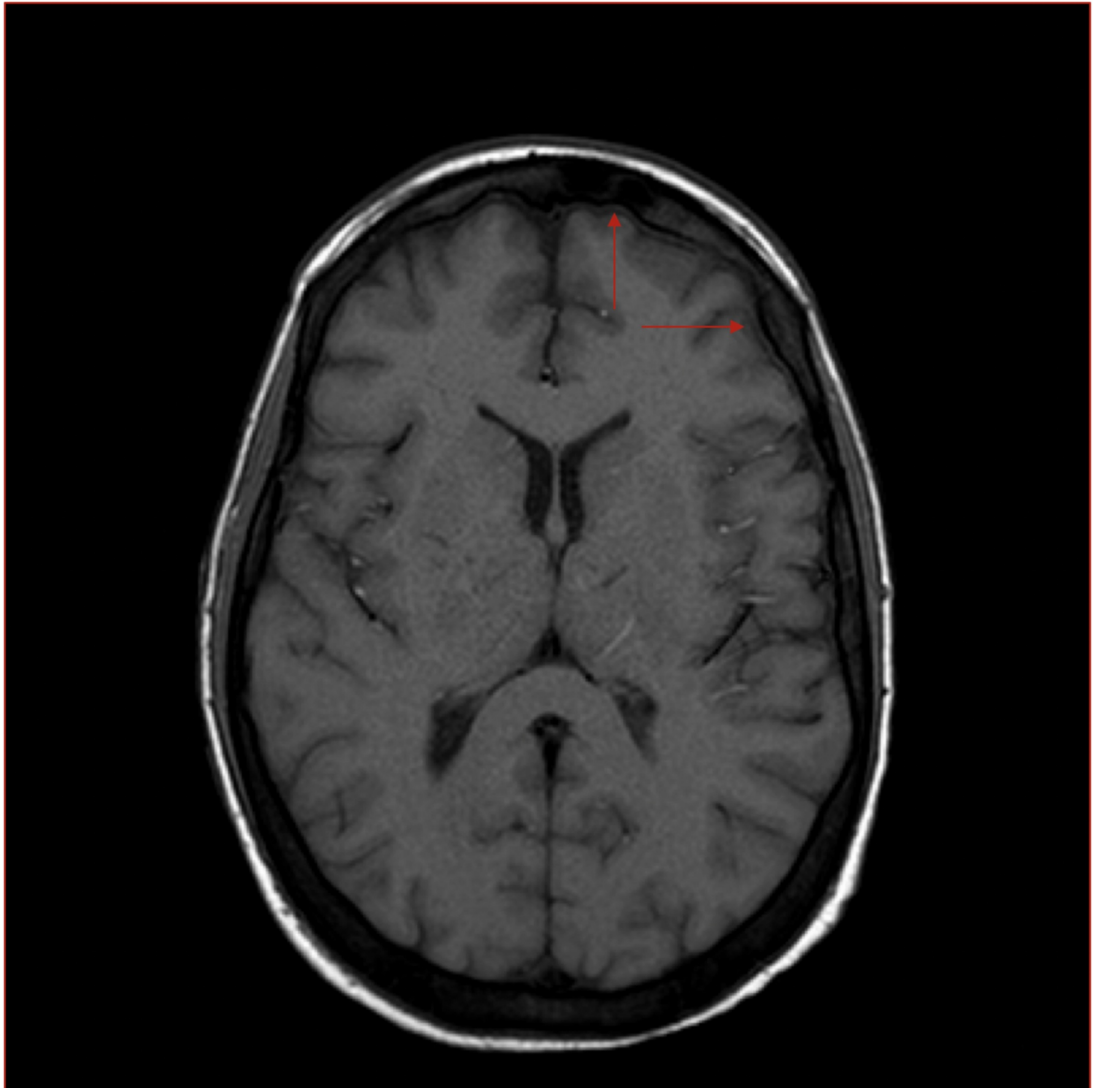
MRI of the brain revealing diffuse leptomeningeal enhancement consistent with meningitis or inflammatory changes
